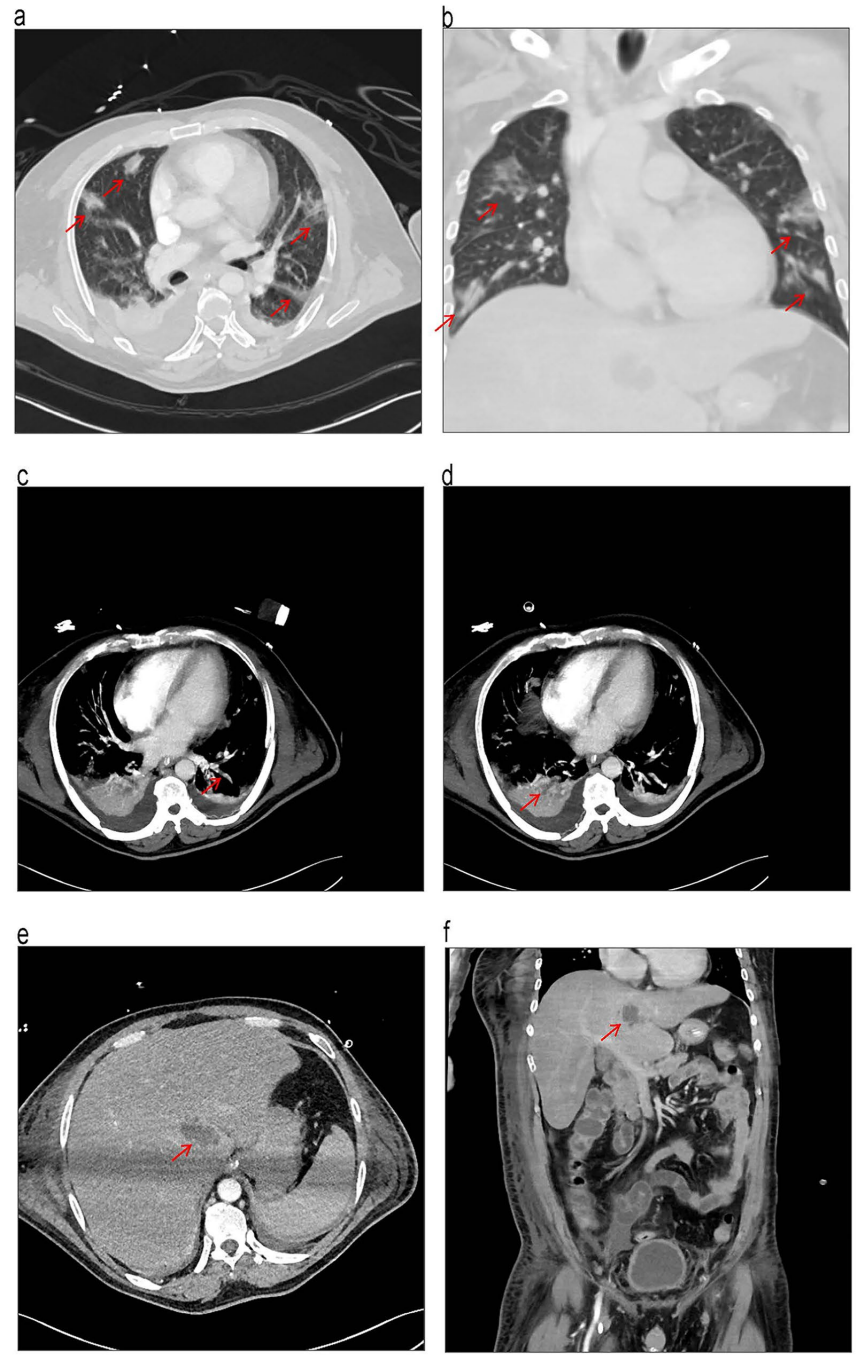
CT imaging findings eight days after admission. (a) and (b) Contrast-enhanced chest CTs reveal an increase in local infection, as indicated by the red arrow. (c) and (d) Improved CT scans suggest the presence of a pulmonary embolism. (e) and (f) Abdominal CT scans show an area of abnormal attenuation in the caudate lobe of the liver, measuring 3.3 cm × 2.1 cm × 2.2 cm
